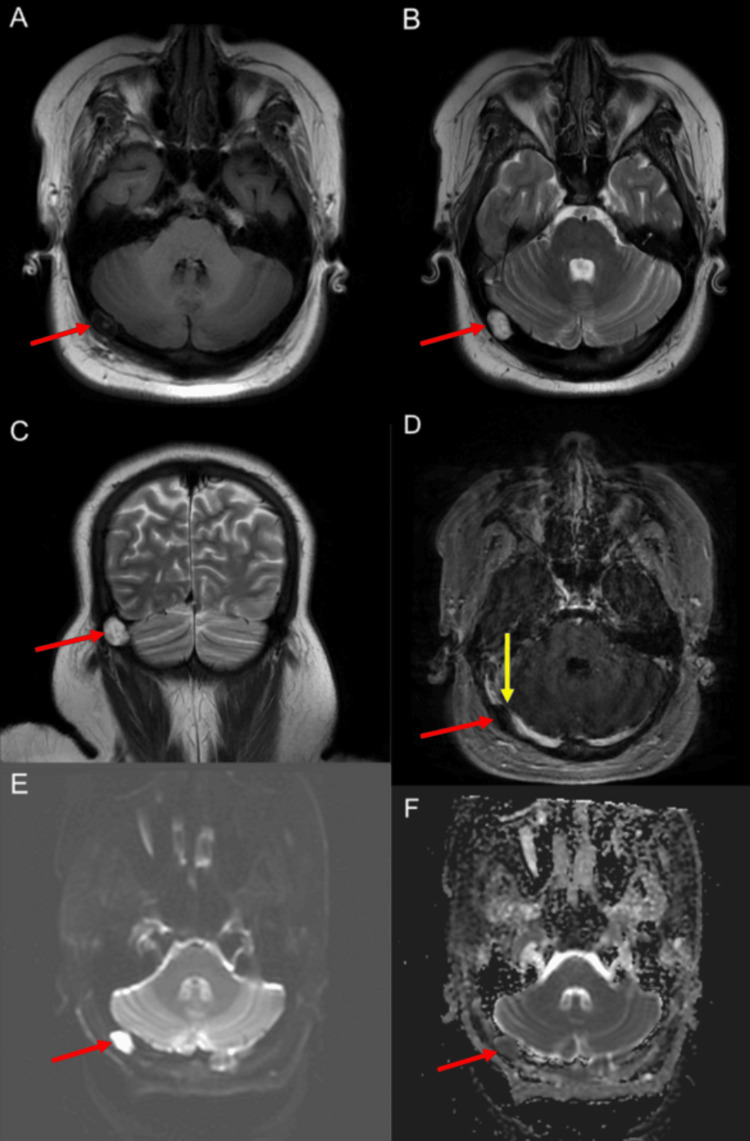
Contrast-enhanced MRI of the brain shows a 1.6 × 1.0 cm lesion within the right parietal/occipital calvarium that is low on T1 and high on T2 (A-C), non-enhancing (D), and diffusion-restricting (E-F). The lesion thins both the inner and outer tables (B, C) and narrows the right transverse sinus (yellow arrow). Imaging characteristics are consistent with an intradiploic epidermoid inclusion cyst (red arrows).
